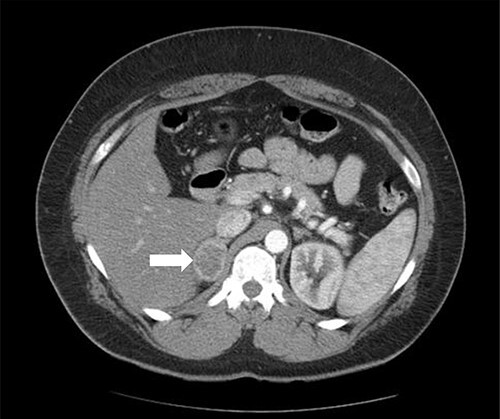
Axial CT of the abdomen showing the right adrenal mass in Patient 1 (white arrow).
